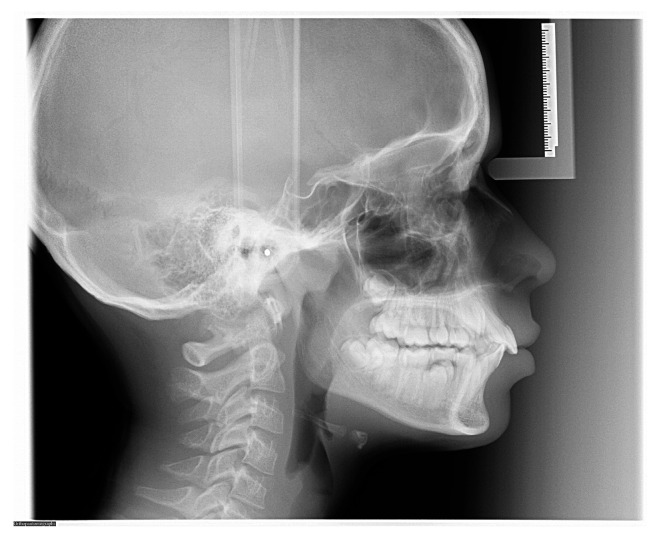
Properly acquired lateral cephalometric radiograph using a direct digital radiography (DR) system with a scanning sensor bar. Patient positioning: standing; Frankfurt plane horizontal; midsagittal plane parallel to the detector; teeth in maximum intercuspation with lips closed. Central ray centered on the external auditory meatus. There is perfect superimposition of the bilateral radiopaque ear rod markers, confirming absence of tilt or rotation. Acquisition parameters: 100 kVp, 20 mA, scanning time ~4 s. The field of view is truncated superiorly and posteriorly, as is typical for DR systems.
